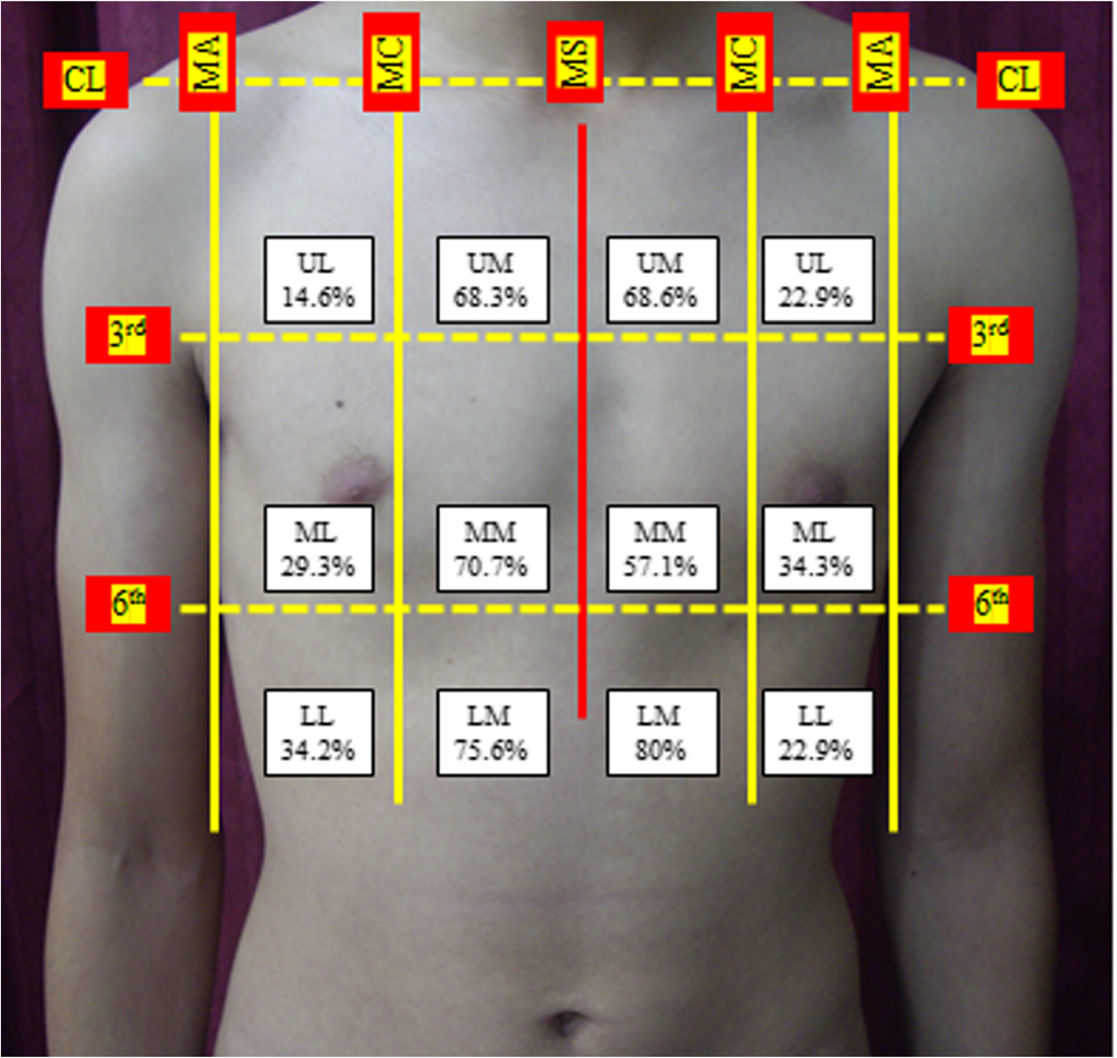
The percentage of air pockets in each hemithorax (right hemithorax 41 patients and left hemithorax 35 patients) according to a grid pattern dividing each hemithorax into six anatomical locations. CL, clavicular line; MC, midclavicular line; MA, midaxillary line; MS, midsternal line; 3rd, 3rd costosternal junction level; 6th, 6th costosternal junction level; UPS, upper parasternal; UL, upper lateral; MPS, middle parasternal; ML, middle lateral; LPS, lower parasternal; LL, lower lateral
